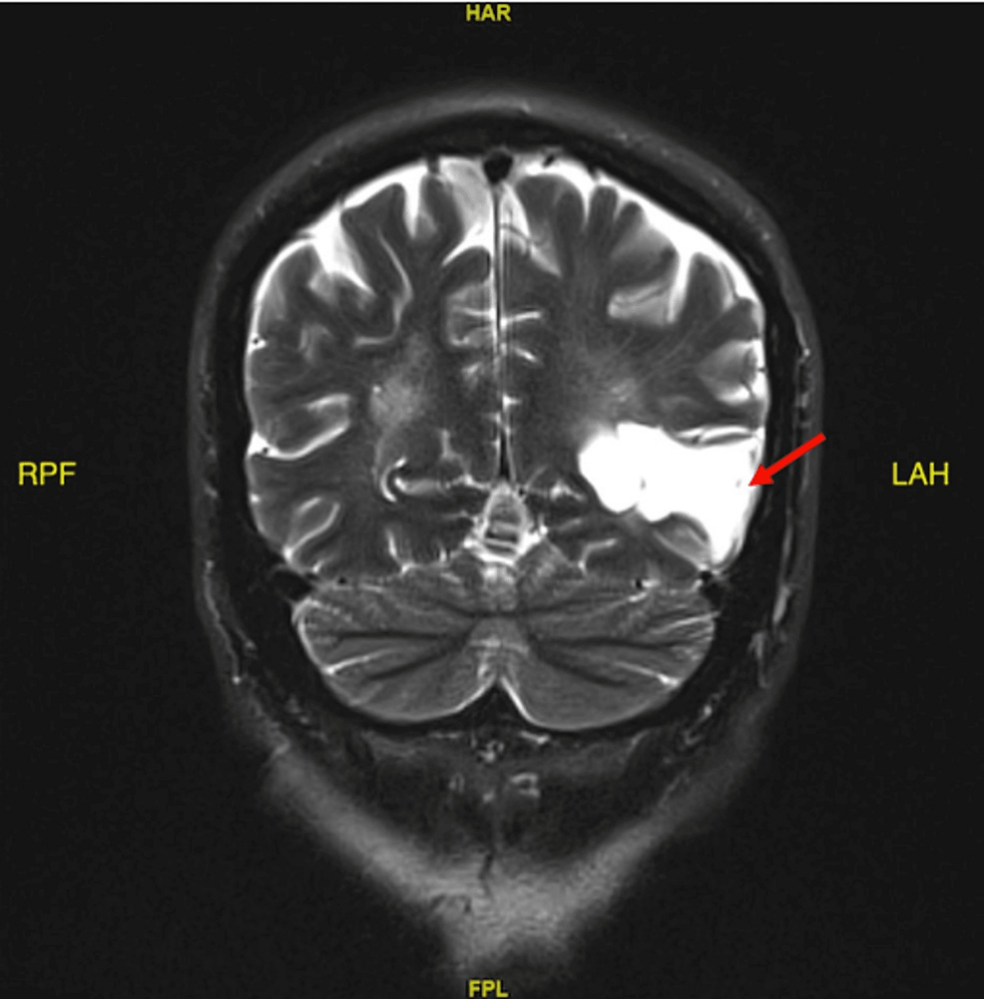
MRI brain with contrast (coronal view), indicating large area encephalomalacia in the left temporoparietooccipital region
